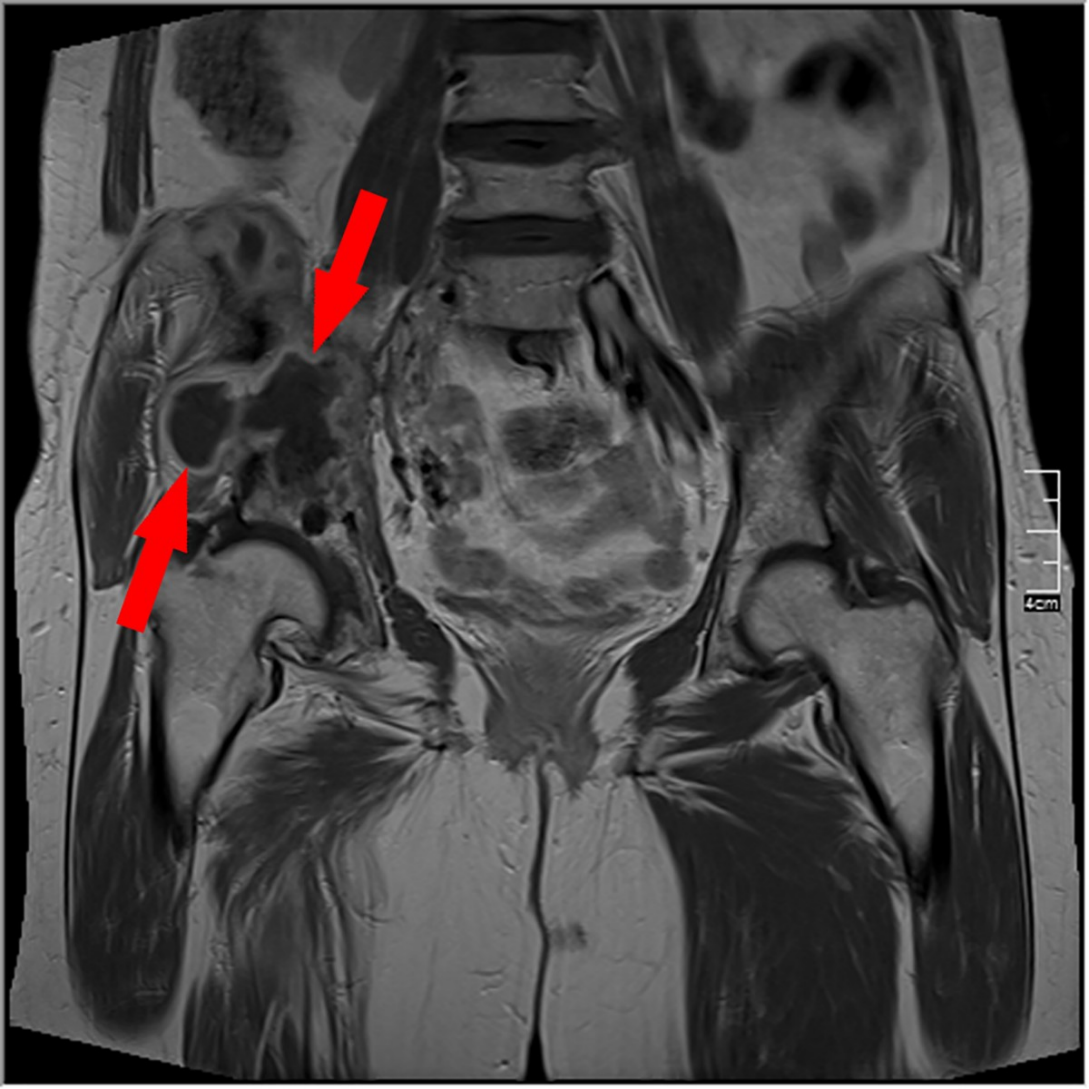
Cystic formation with intra- and extrapelvic parts as well as migration of the right Ala ossis ilii and sacroiliac joint (T1 post-contrast agent). *Image credit*: *S*. *Gießler*. T1, T1-weighted imaging.
